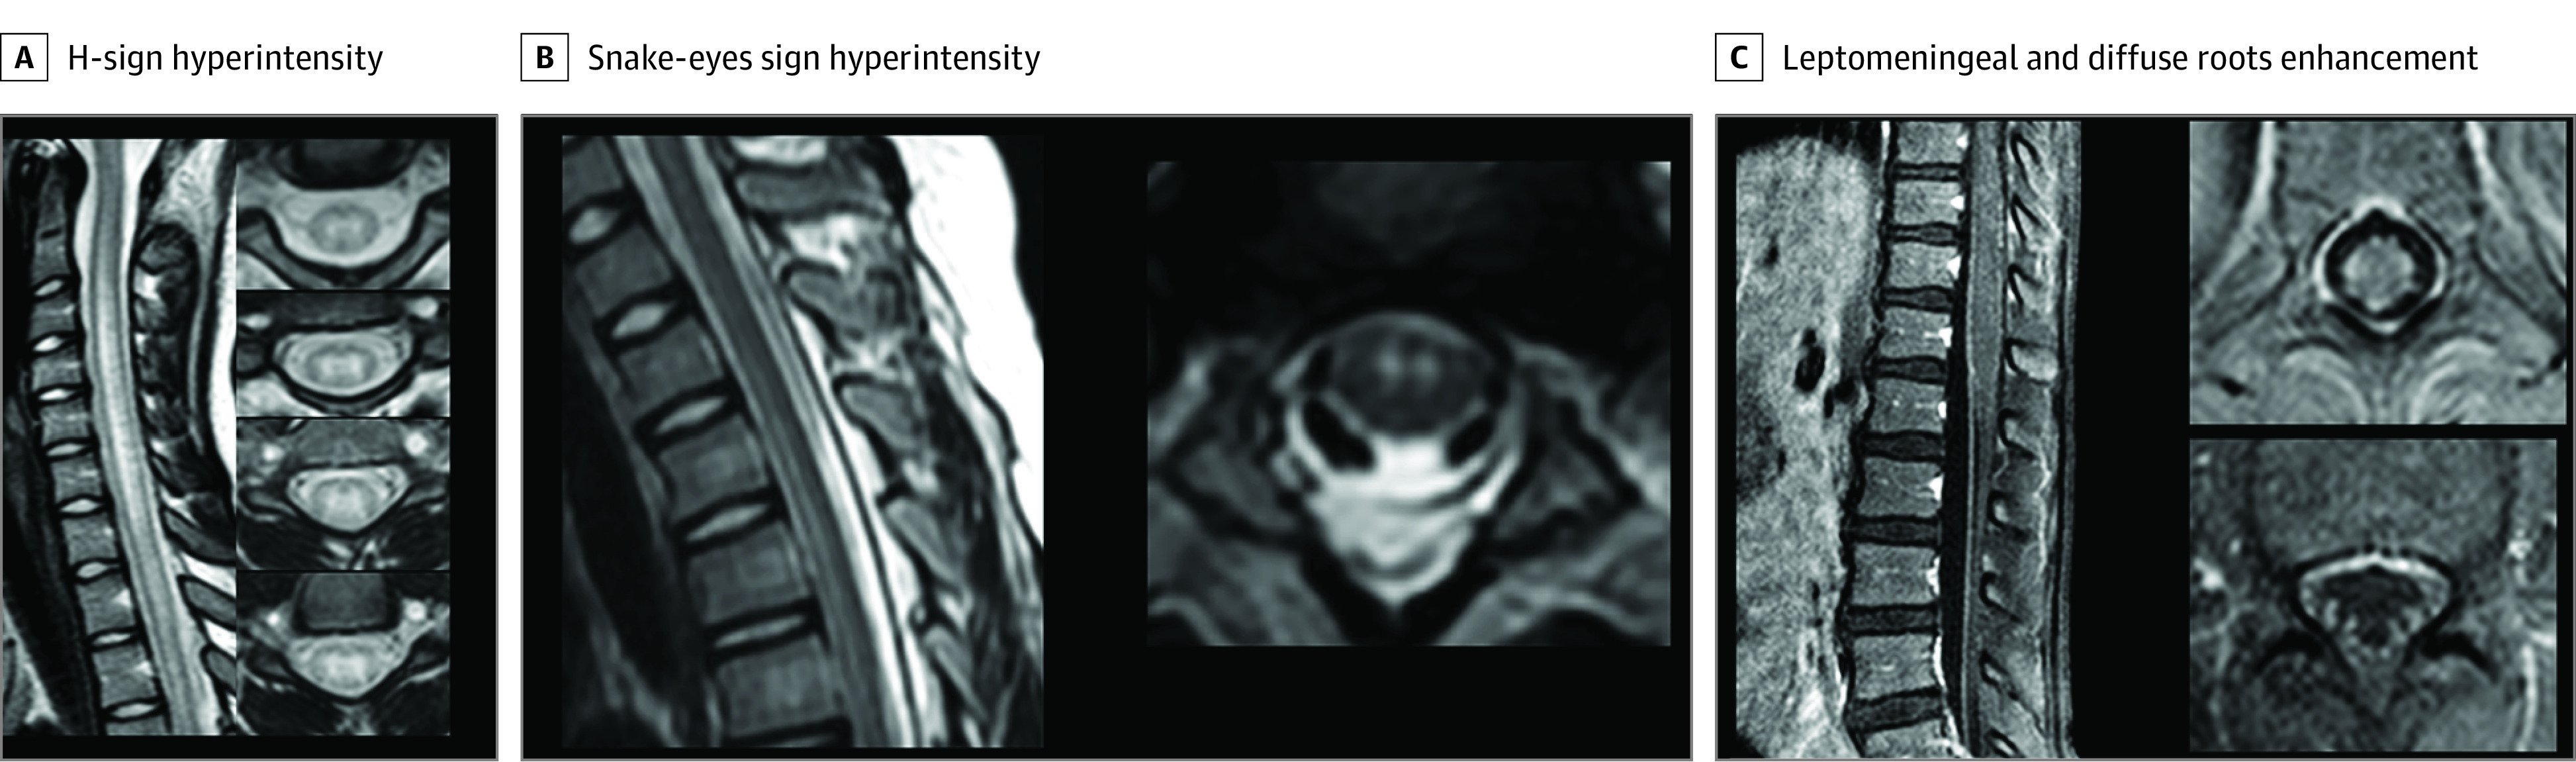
Characteristic Imaging Features of Spinal Cord Involvement in Myelin Oligodendrocyte Glycoprotein Antibody–Associated Disease A, Sagittal (left) and axial (right) T2-weighted magnetic resonance imaging (MRI) of the spine shows a spinal cord lesion spanning over more than 10 vertebral segments, with H-sign appearance most evident in the cranial and caudal extremities of the lesion and hazy involvement of most of the cross-sectional spinal cord area in the central portion of the lesion. B, Sagittal (left) and axial (right) T2-weighted MRI of the spine shows a longitudinally extensive lesion with pencil-sign appearance on sagittal view and corresponding anterior-horns hyperintensity on axial image. C, Sagittal (left) and axial (right) gadolinium-enhanced T1-weighted MRI of the spine shows leptomeningeal and diffuse roots enhancement.
